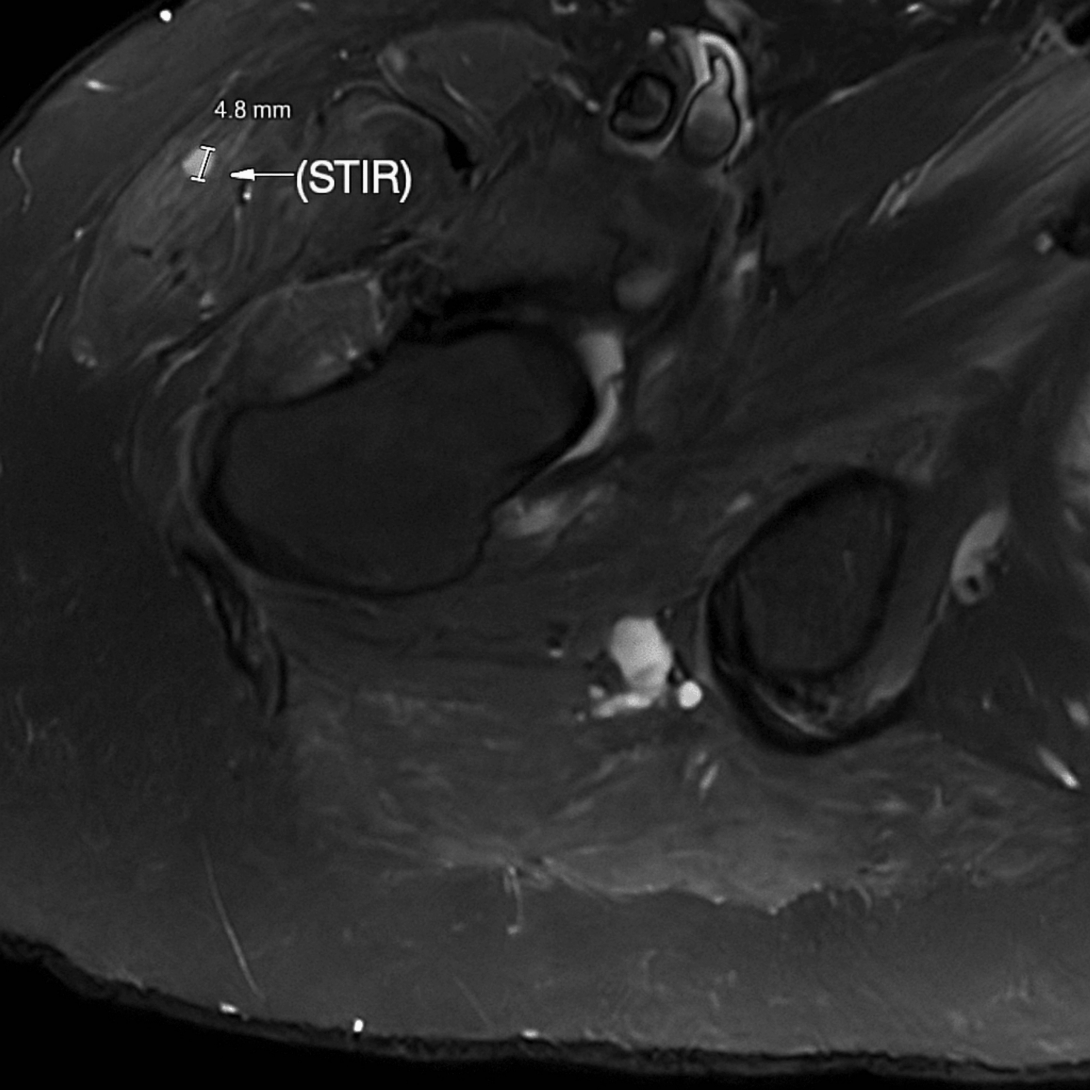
Axial STIR MRI of the right thigh Arrow showing diffuse hyperintensity and edema in the adductor muscles, consistent with inflammatory myopathy. STIR, short-tau inversion recovery
